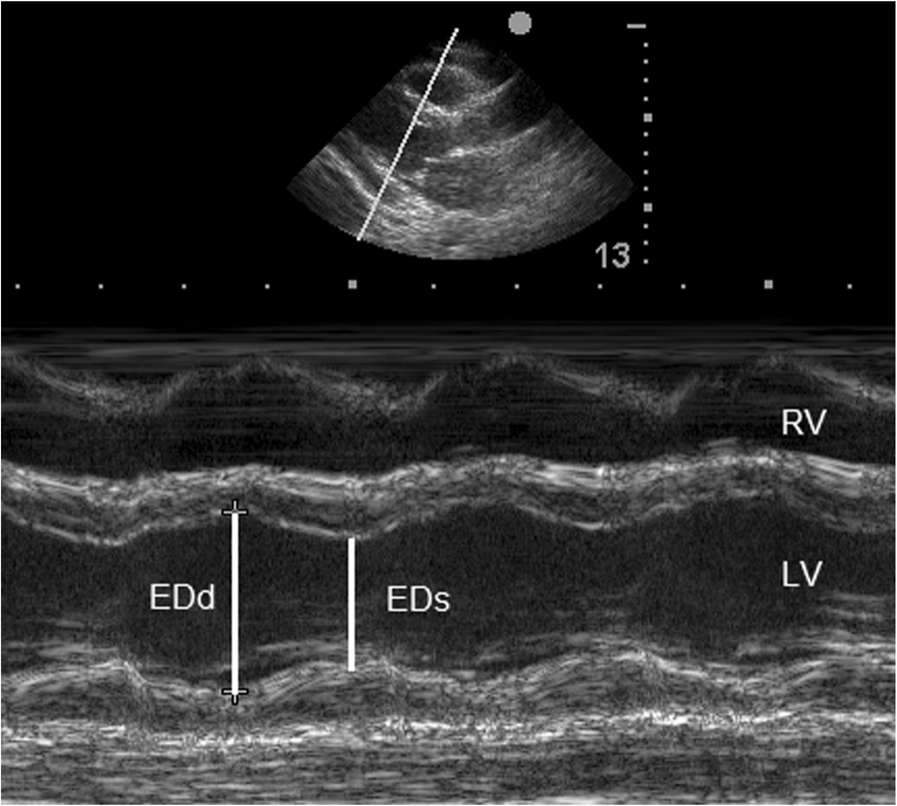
Measurement of the ejection fraction using M-mode in the parasternal long-axis view. The LV end-diastolic internal dimensional (EDd) is measured at the largest dimension, and the LV end-systolic internal dimensional (ESd) is measured at the smallest dimension. RV, right ventricle.
